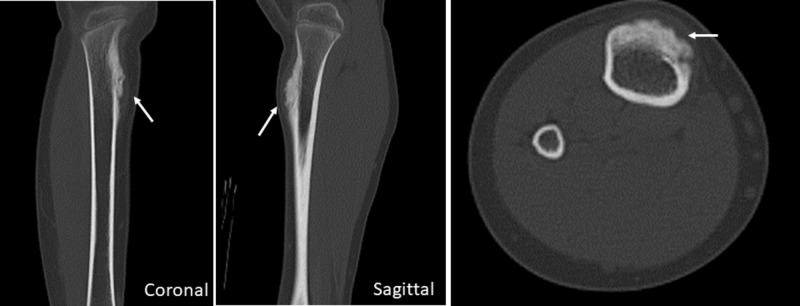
Computed tomography (CT) of the tibial periosteal osteosarcoma before neoadjuvant chemotherapy (NAC) Pre-neoadjuvant chemotherapy (NAC) non-enhanced coronal and sagittal two-dimensional reconstruction images and axial image with bone setting of the lower leg show a juxtacortical mass with thick calcification and periosteal reaction.
